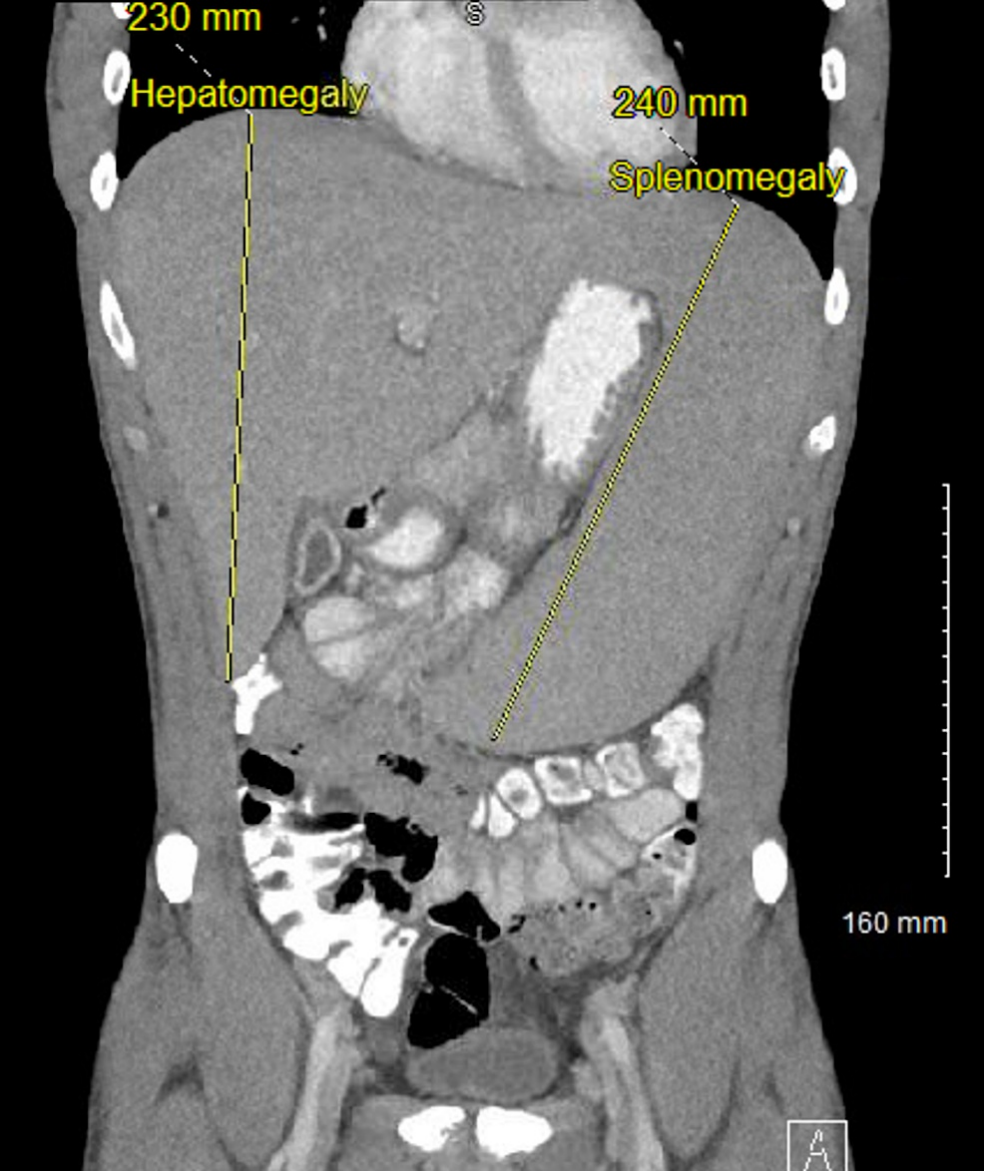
Contrast-enhanced CT abdomen and pelvis (coronal view) demonstrating hepatosplenomegaly.
